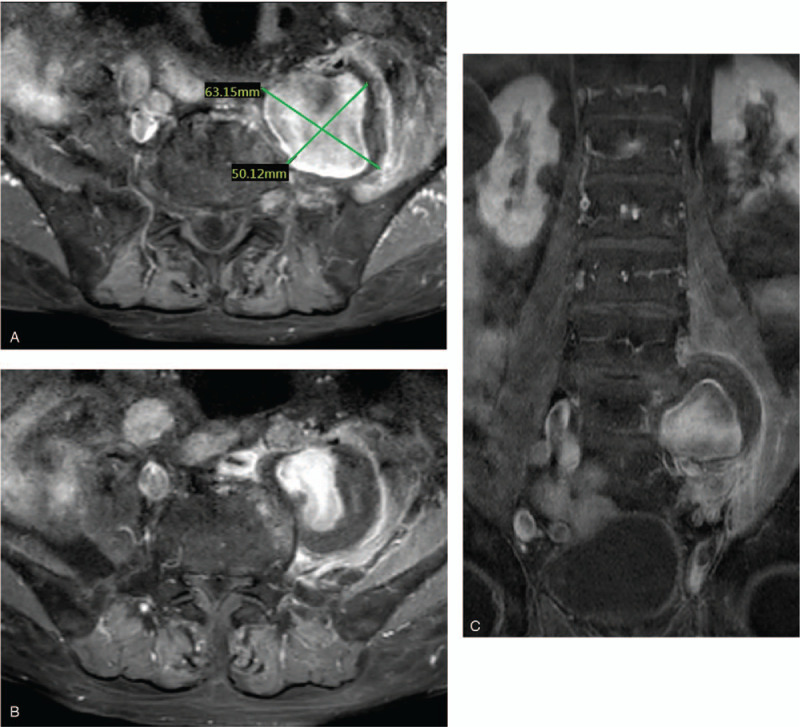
Magnetic resonance imaging (MRI) exam for spinal cord lesion: (A) a common iliac artery aneurysm compressed the psoas muscle, at 63 mm in diameter. (B) The aneurysm was from common iliac. (C) The aneurysm was fusiform; the internal and external iliac artery were patent without aneurysm changes.
